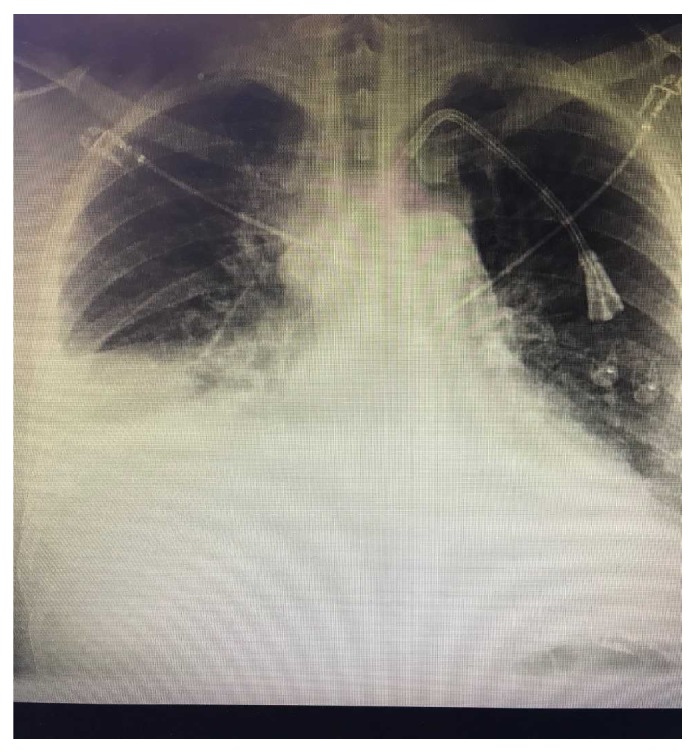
Initial chest x-ray demonstrating pleural effusion and abnormal cardiac silhouette.
